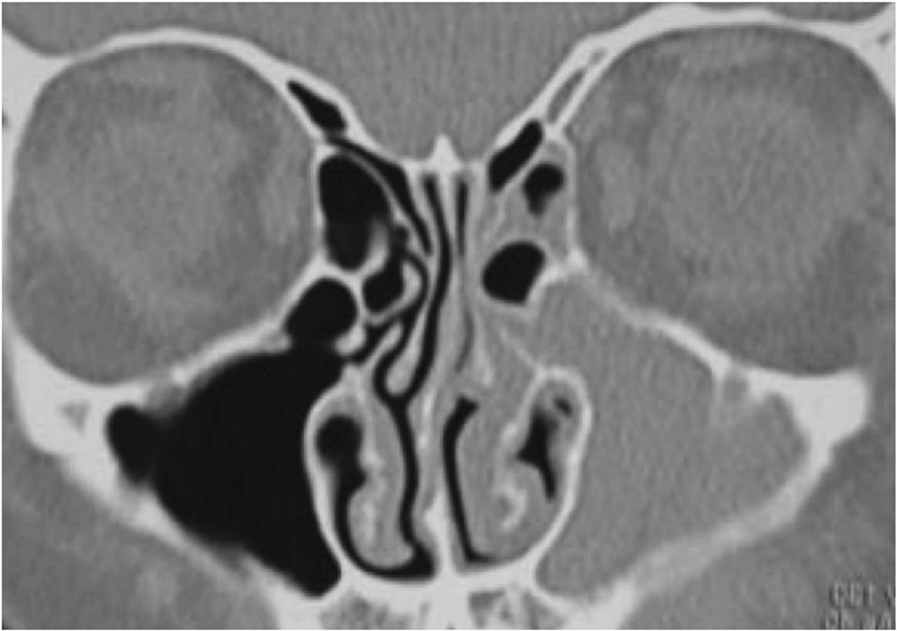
Coronal CT scan through the paranasal sinuses demonstrating extensive nasal polyposis.
